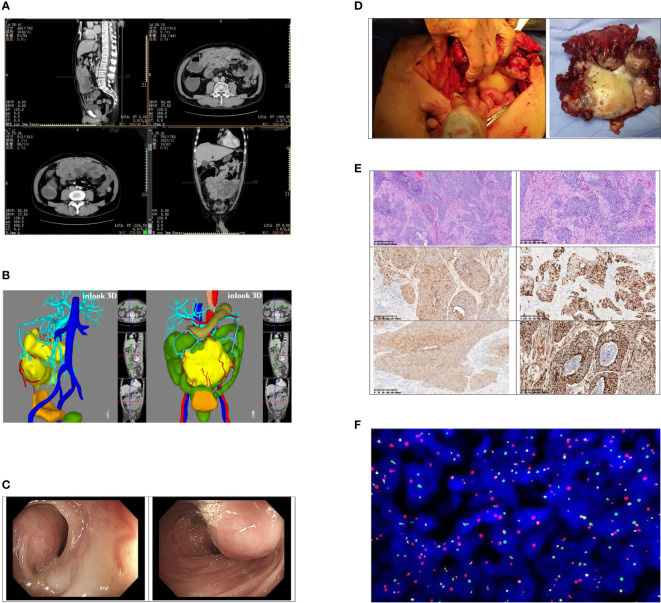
(A) Contrast-enhanced CT found that the larger cross-section range is about 167*149*65 mm; moderate uneven enhancement occurred after the enhancement; obscure boundary, unresolved from the adjacent transverse colon and part of the small intestine. Multiple effusion dilations were seen in the ascending colon and part of the small intestine. (B) The position of the tumor in the abdominal cavity and its relationship with various organs were displayed by 3D reconstruction imaging of CT. (C) A huge external pressure mass under the mucosa at the middle section of the transverse colon (approximately 65 cm away from the anus) made the intestinal lumen compressed, twisted, and narrow. (D) The tumor involved the middle part of the transverse colon and part of the bladder wall. The size was about 18 * 12 * 6 cm. (E) The pictures of tumor pathology sections; order is: ×40, ×100, CKpan (+), desmin (+), NSE (+), vim (+). (F) Project name: EWSR1/WT1 fusion gene test (FISH-tissue). Detection probe: LBPEWSR1/WT1 fusion gene probe. Chromosome loci: WTl (llpl3)/EWSRl (22ql2). Probe tag: green signal is GSPEWSR1, red signal (R) is the GSPWT1 detection result. Cell number analyzed: 200; Diagnostic opinion: The EWSR1/WT1 fusion gene was detected, 200 interval phase cells were analyzed, and each signal pattern was as follows: 1G1R1F39.0%, 1G1R2F20.0%, 1G1F4.0%, lG2F 5.0%, lRlF 5.0%, lR2F 4.0%, lGlR 9.0%, 2GlR 5.0%, lG2R 4.0%, 2G2R 5.0%.
